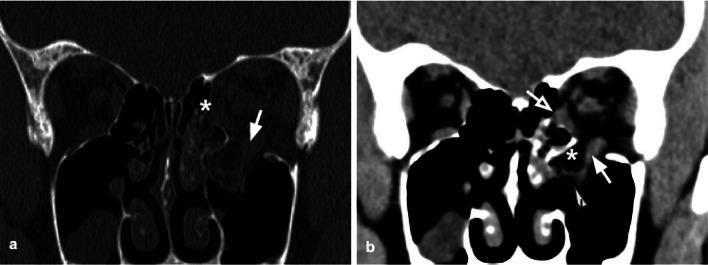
Coronal CT reconstruction with bone (a) and soft tissues (b) algorithms of an orbital floor fracture associated with a lamina papyracea fracture. a Left orbital floor fracture with huge bone defect (arrow), associated with medial wall fracture (white asterisk). b Herniation of the intraorbital soft tissues into the maxillary sinus (white asterisk). The inferior rectus muscle is dysmorphic, elongated, and also partially herniated caudally into the sinus (arrow). In the lower portion of the herniated tissue, a small hematoma is present (arrowhead). The medial rectus muscle (white asterisk) is swollen and partially entrapped in the fracture of the lamina papyracea (empty arrow)
